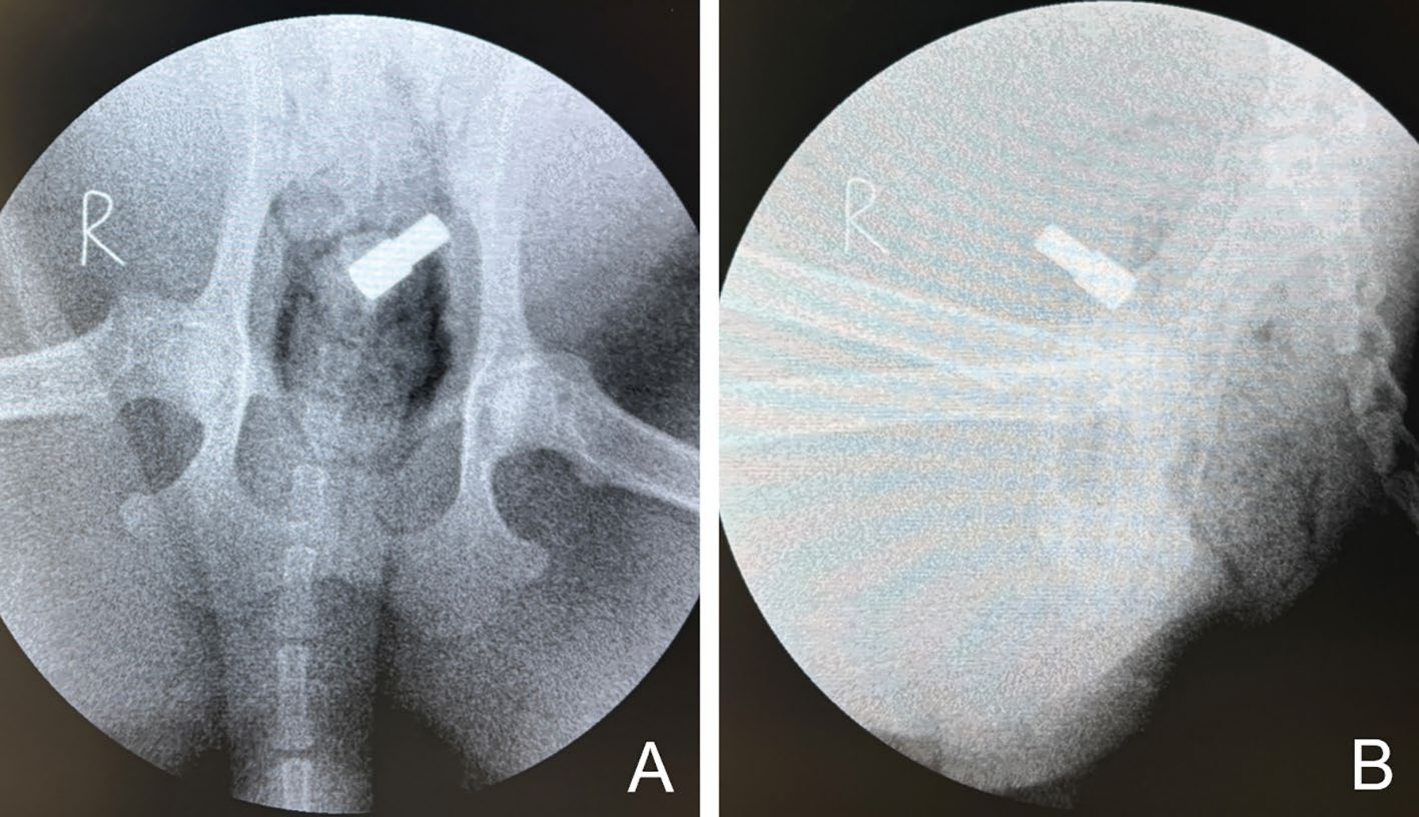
X-ray examination performed 2 weeks postoperatively showed the location of the magnet. (A) Ventrodorsal radiograph. (B) Lateral radiograph.
